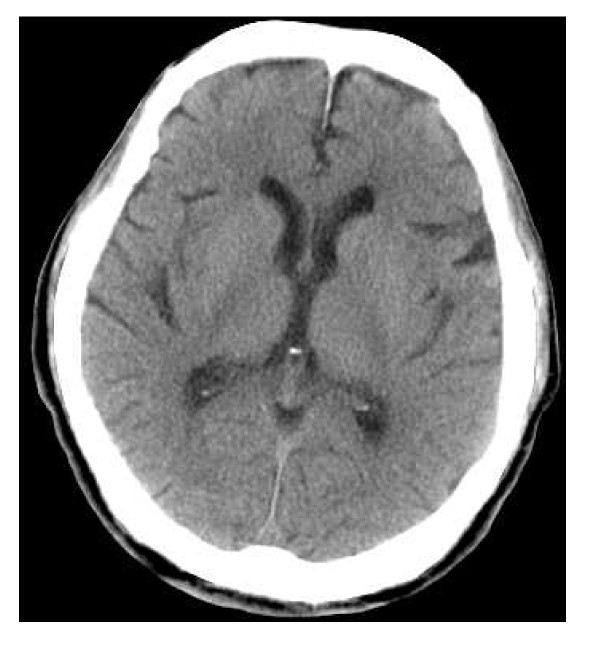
Head computed tomography on admission.
